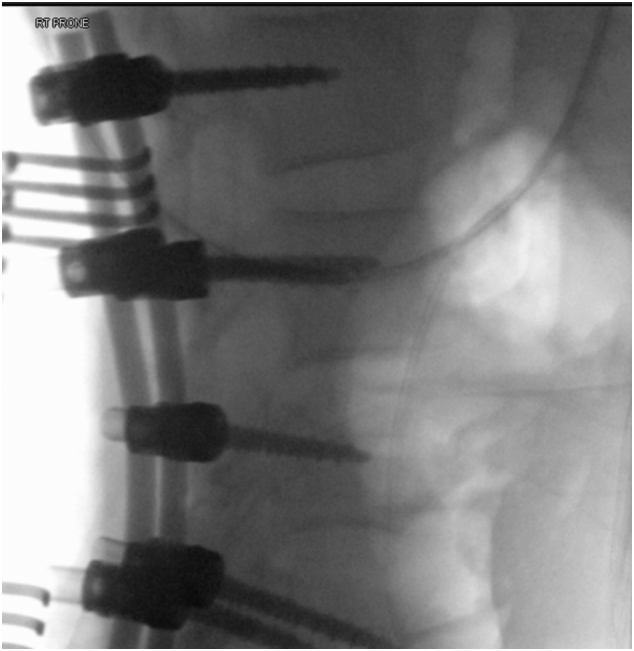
Intraoperative radiograph shows posterior stabilisation from L1 to L5.
